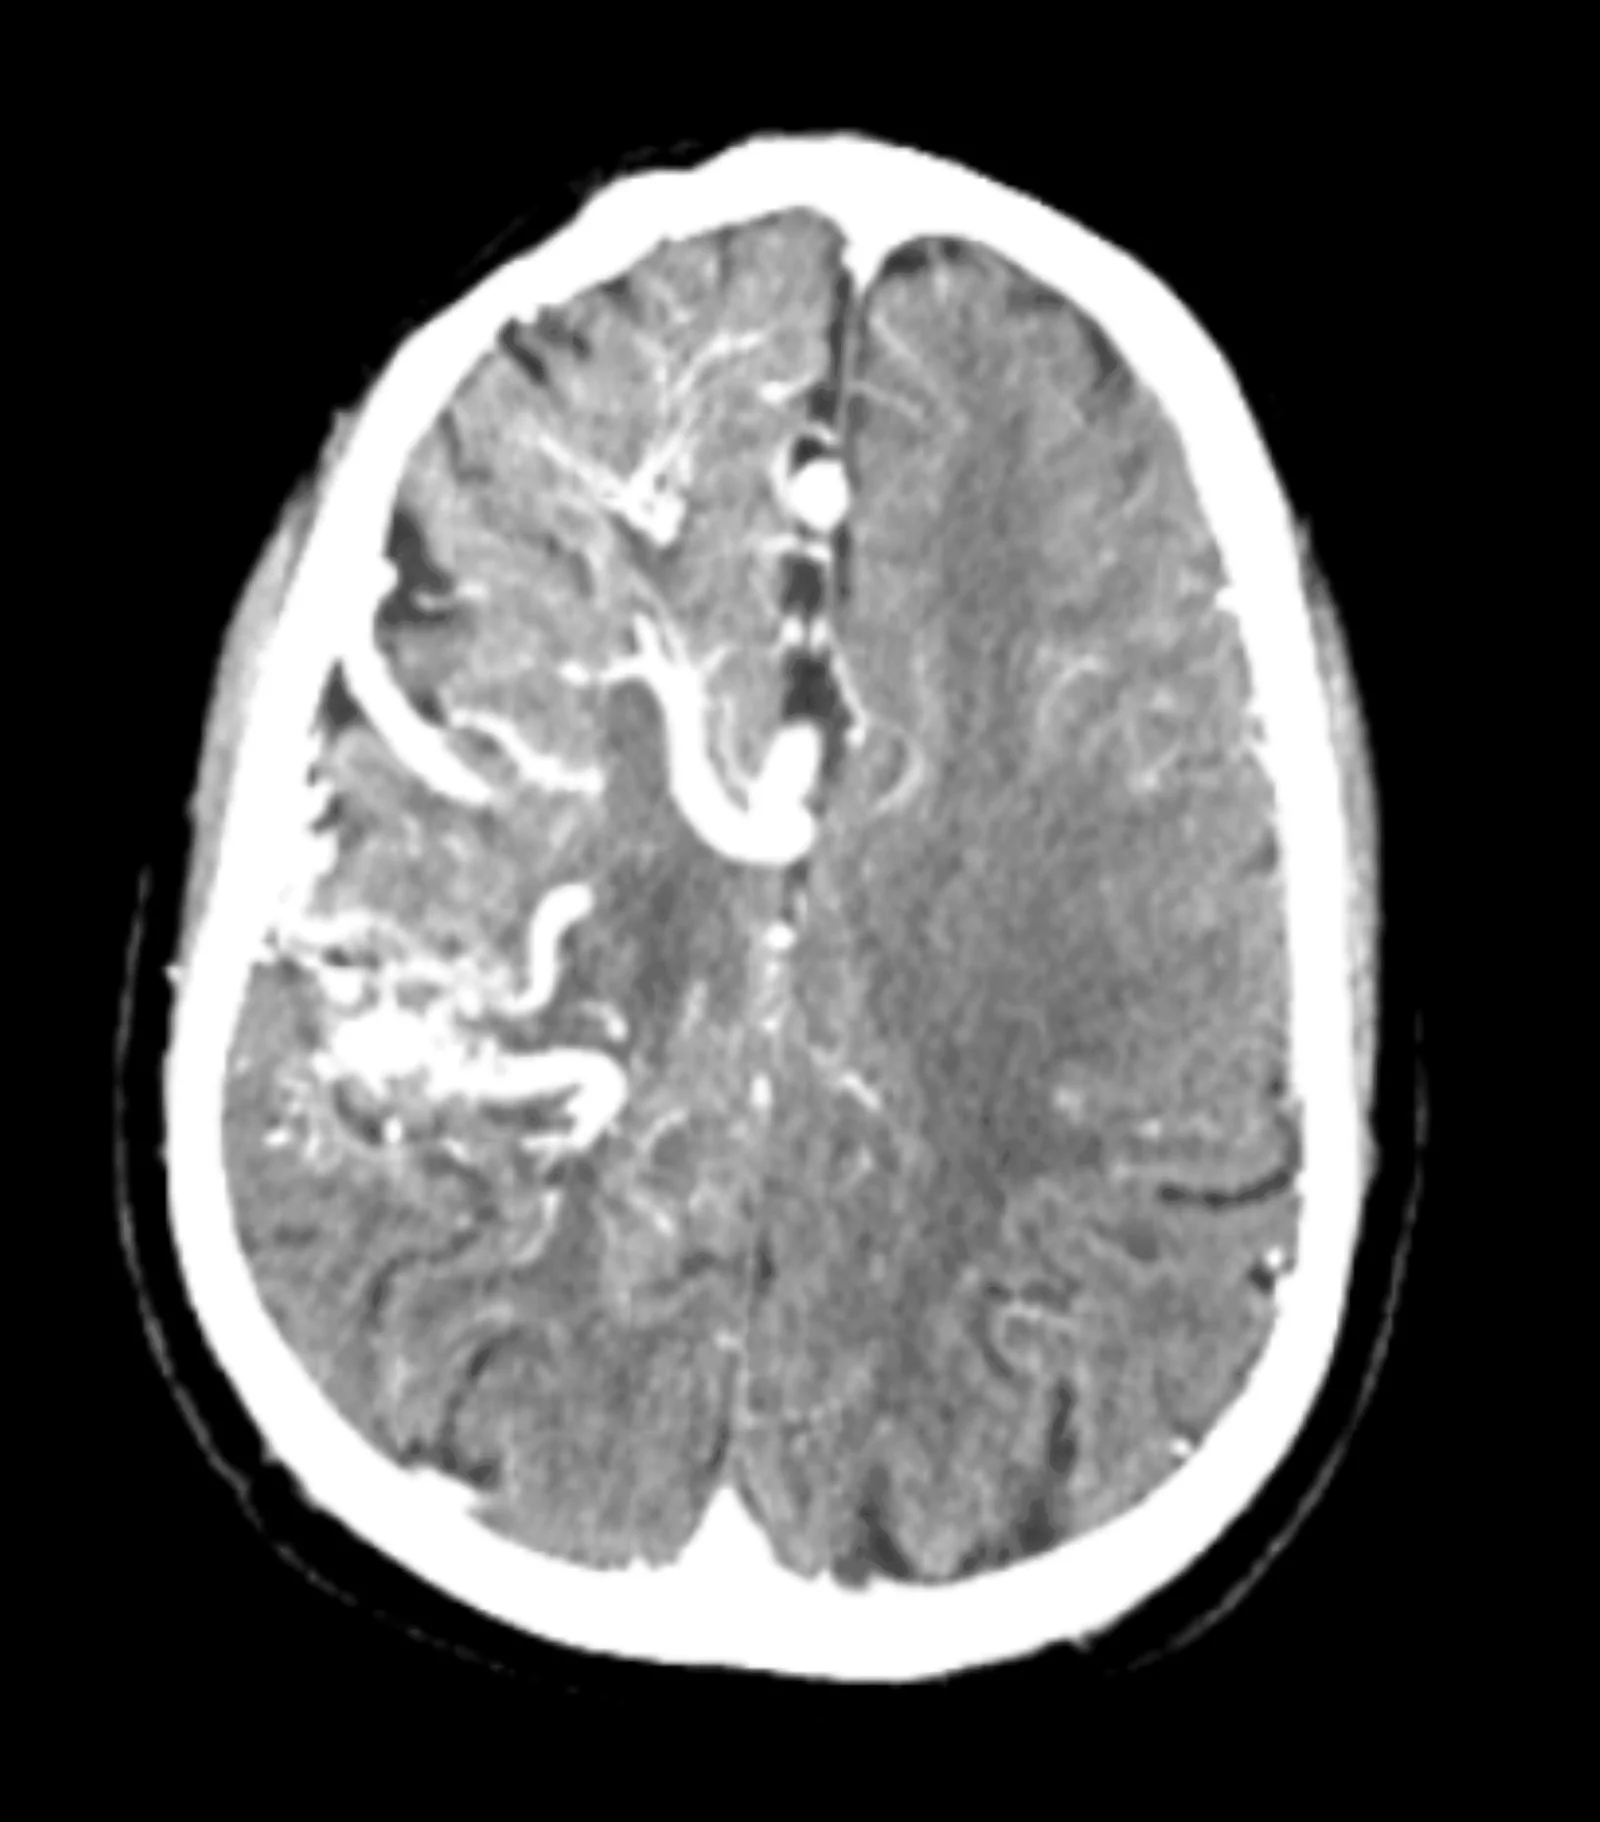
Follow-up contrast-enhanced CT scan, axial section, demonstrating stable appearance of the vascular malformations and the developmental venous anomaly (DVA), with no evidence of intracranial hemorrhage
